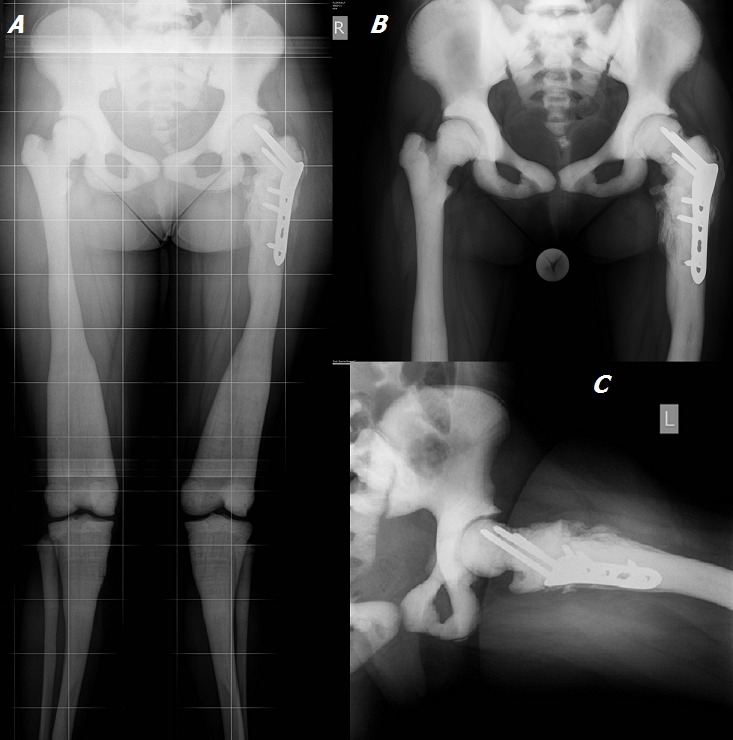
X-rays on 1 year post-operatively. Fracture consolidation, femoral neck-shaft angle of 132°, no lower limb length discrepancy
